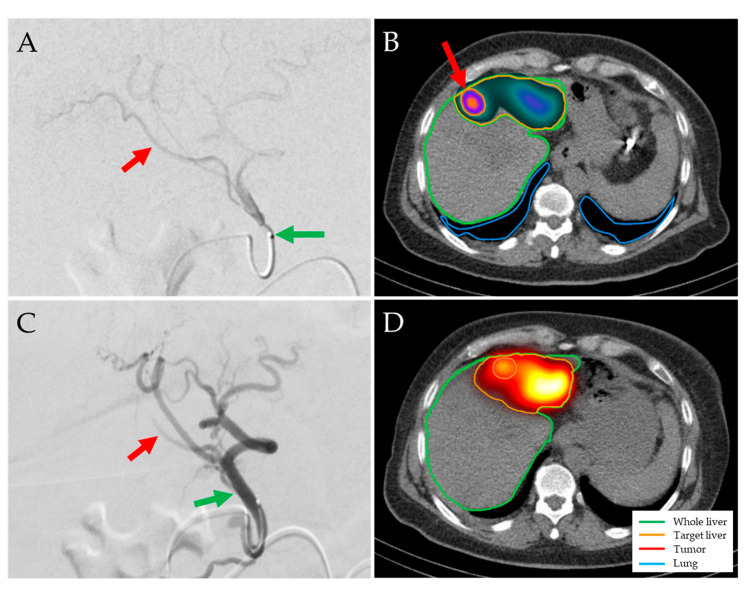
Discrepancy between planning and treatment procedures in a 65-year-old woman with HCC stage IIIA. QuiremScout microspheres were injected into the left hepatic artery (A, green arrow). SPECT/CT showed satisfactory activity deposition in the tumor in the liver segment IVa (B, red arrow; planned tumor/healthy target doses of 164 Gy/55 Gy). A total of 0.8 GBq QuiremSpheres microspheres were injected (C). Post-therapeutic imaging showed a different microsphere distribution with higher activity in non-tumor tissue (D, mean tumor/healthy target doses of 86 Gy/57 Gy), possibly due to a slightly more distal catheter position and a segment IV branch (A,C, red arrows) arising distally but opposite to the microcatheter tip (C, green arrow).
